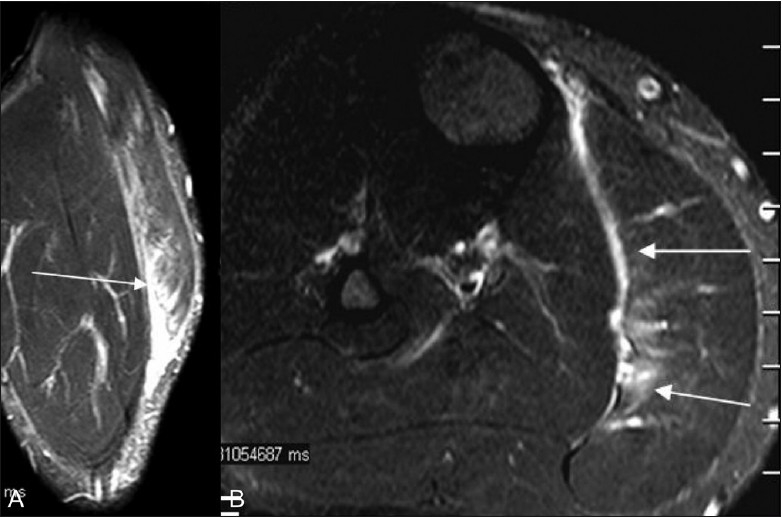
MRI. Coronal T2W (A) and axial fat-saturated T2W (B) images show hyperintensity of the gastrocnemius muscle due to a partial tear (arrows) with fluid along the triceps surae
